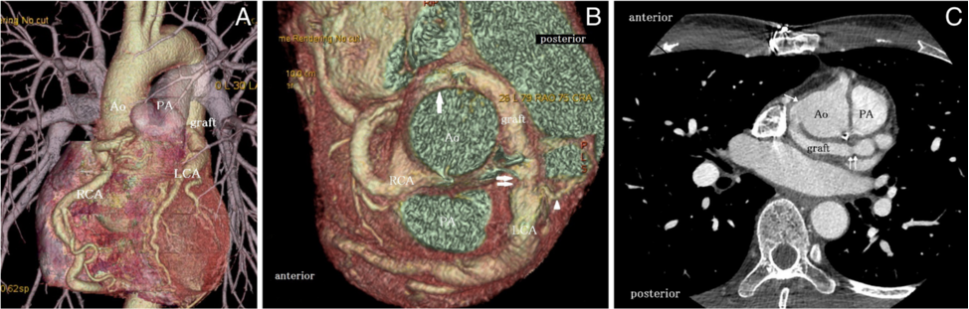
Computed tomography exam results in different perspectives. (A) Multidetector-row computed tomography 2 years after surgery. The diameter of the preoperatively dilated coronary artery reduced and the collaterals disappeared. (B) Cranial view on multidetector-row computed tomography at 2 years after surgery. The arrow indicates that the graft was anastomosed to the right lateral side of the ascending Ao. The double arrows indicate that the graft was anastomosed to the LCA. The arrowhead indicates the collateral located adjacent to the anastomotic site of the graft and the LCA. (C) Axial image on enhanced computed tomography at 2 years after surgery. The arrow indicates that the graft was anastomosed to the right lateral side of the ascending Ao. The double arrows indicate that the graft was anastomosed to the left coronary cuff. Ao, aorta; PA, pulmonary artery; RCA, right coronary artery; LCA, left coronary artery. Graft: an 8-mm HEMASHIELD graft (MAQUET Cardiovascular LLC, San Jose, CA, USA).
